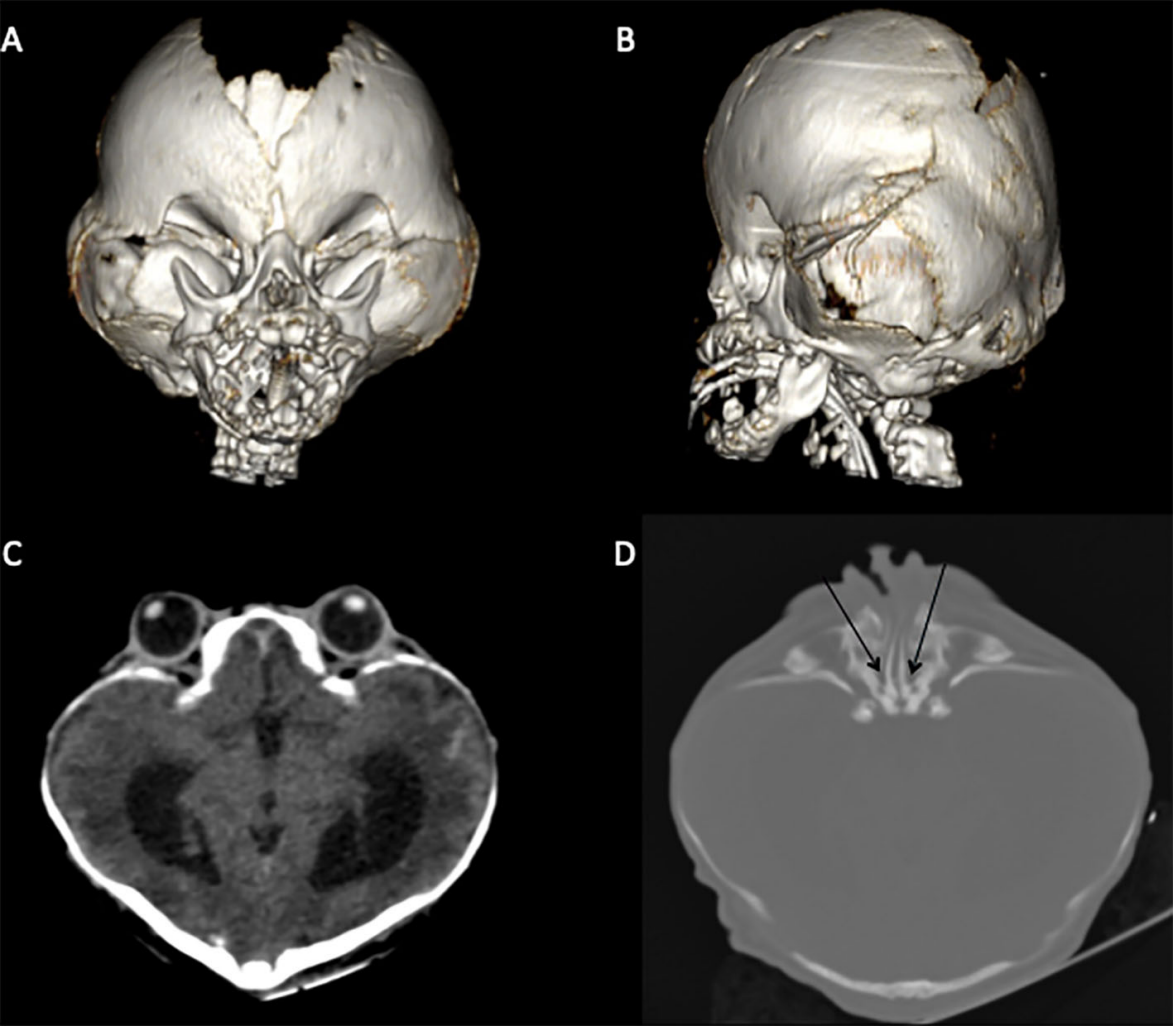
CT scan highlighting classical BSS morphological characteristics. (A, B) brachycephaly due to premature fusion of coronal and lambdoid sutures, orbital deformity, and midface hypoplasia. (C) Hyperteleorbitism and clover-leaf cranial shape. (D) Choanal atresia (black arrows).
